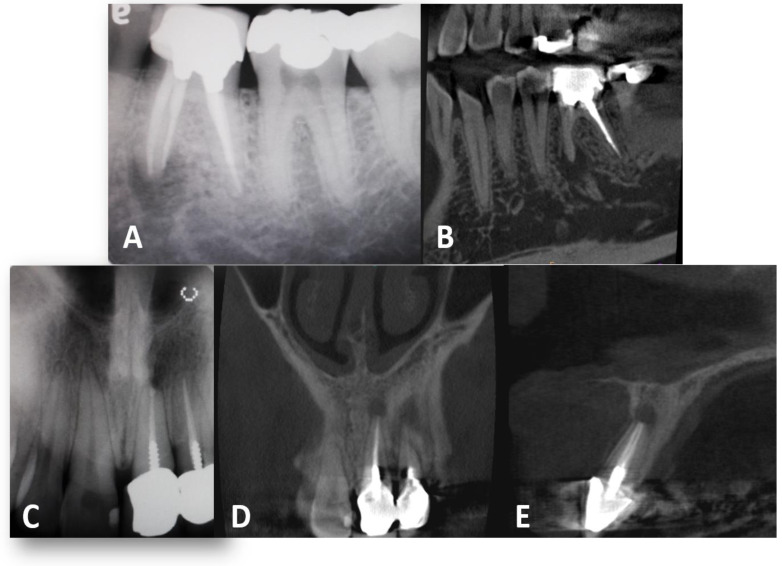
(A) A PA radiography of the first mandibular molar: post was not identified, there are amalgam dowels. (B) Frontal section from CBCT imaging of the same tooth. The observers identified a prefabricated post. (C) A PA radiography of the central incisor: a prefabricated was identified. (D,E) frontal and sagittal sections from CBCT imaging of the same tooth. The observers identified a cast post.
